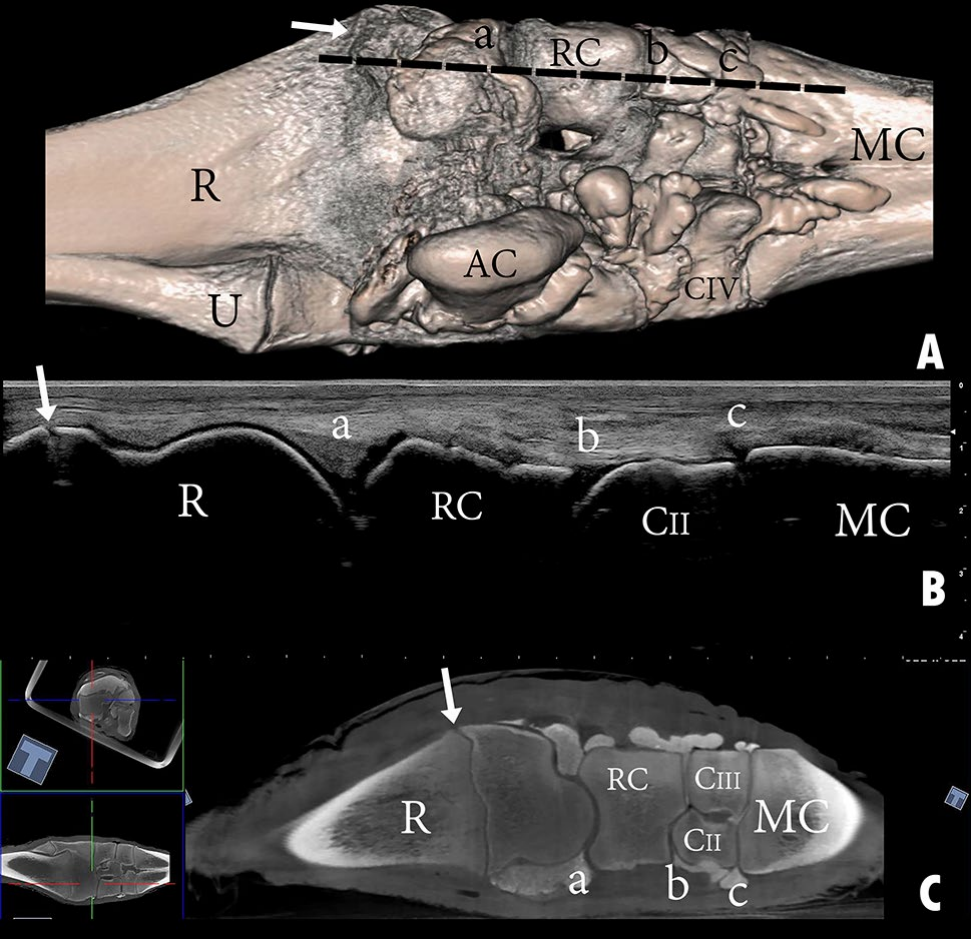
Palmar view of a left carpal joint showing (A) three-dimensional reconstruction of the carpal joint (the black dotted line indicates the palmomedial placement of the ultrasound probe); (B) longitudinal composed ultrasound image of the carpal joint; (C) sagittal plane computerized tomography image. R, the distal end of the radius; U, the distal end of the ulna; AC, accessory carpal bone; RC, radiocarpal bone, CII, carpal bone II; CIII, carpal bone III; CIV, carpal bone IV; MC, metacarpal bone; a, radiocarpal joint cavity; b, intercarpal joint cavity; c, carpometacarpal joint cavity. The white arrow indicates the epiphysial line.
